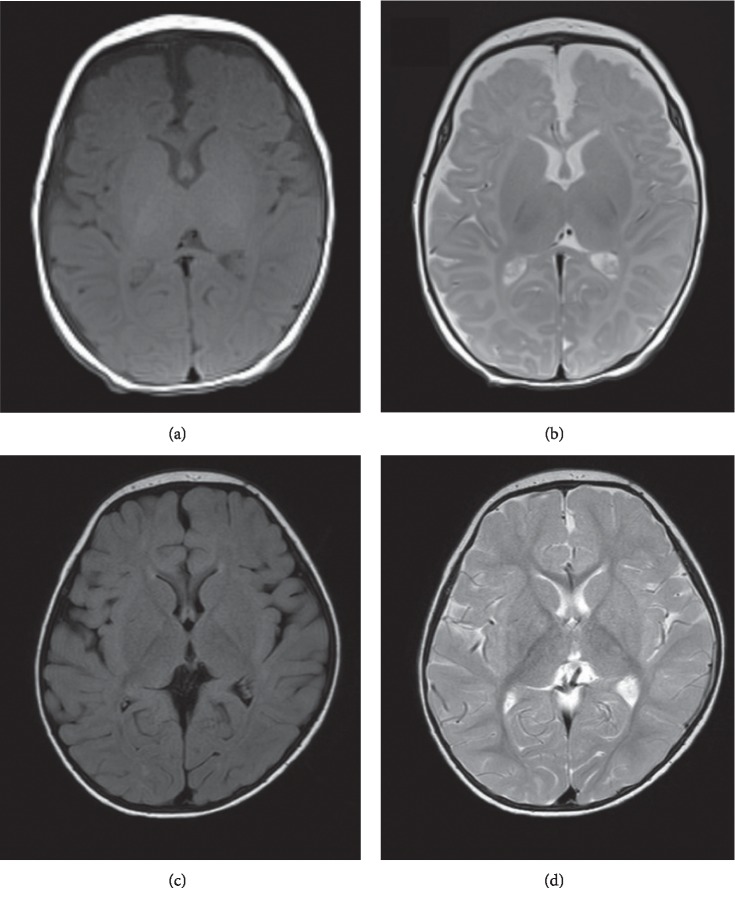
Brain magnetic resonance imaging in the patient with severe propionic acidemia. (a) T1-weighted imaging at the age of 2 months, (b) T2-weighted imaging at the age of 2 months, (c) T1-weighted imaging at the age of 23 months, and (d) T2-weighted imaging at the age of 23 months.
